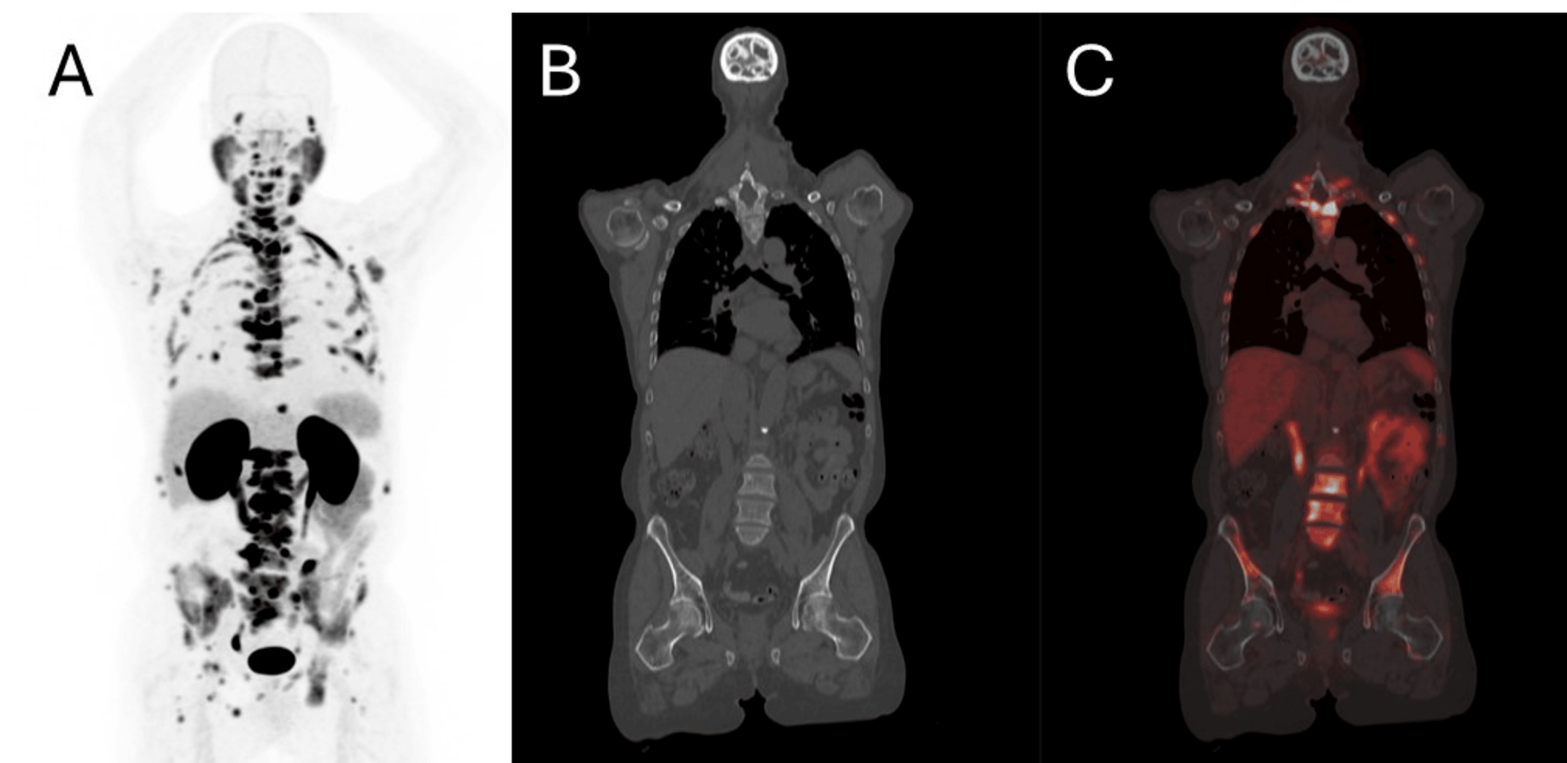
PET before Lu-177 vipivotide tetraxetan. Pre-therapy imaging. A. 3D PET image; B. Coronal CT image; C. Fused PET/CT image. Baseline diagnostic imaging with Ga-68 prostate-specific membrane antigen-11 positron-emission tomography/computed tomography (Ga-68 PSMA-11 PET/CT) revealed widespread bone metastases within the axial and appendicular skeleton (dark areas in A; bright thermal areas in C).
